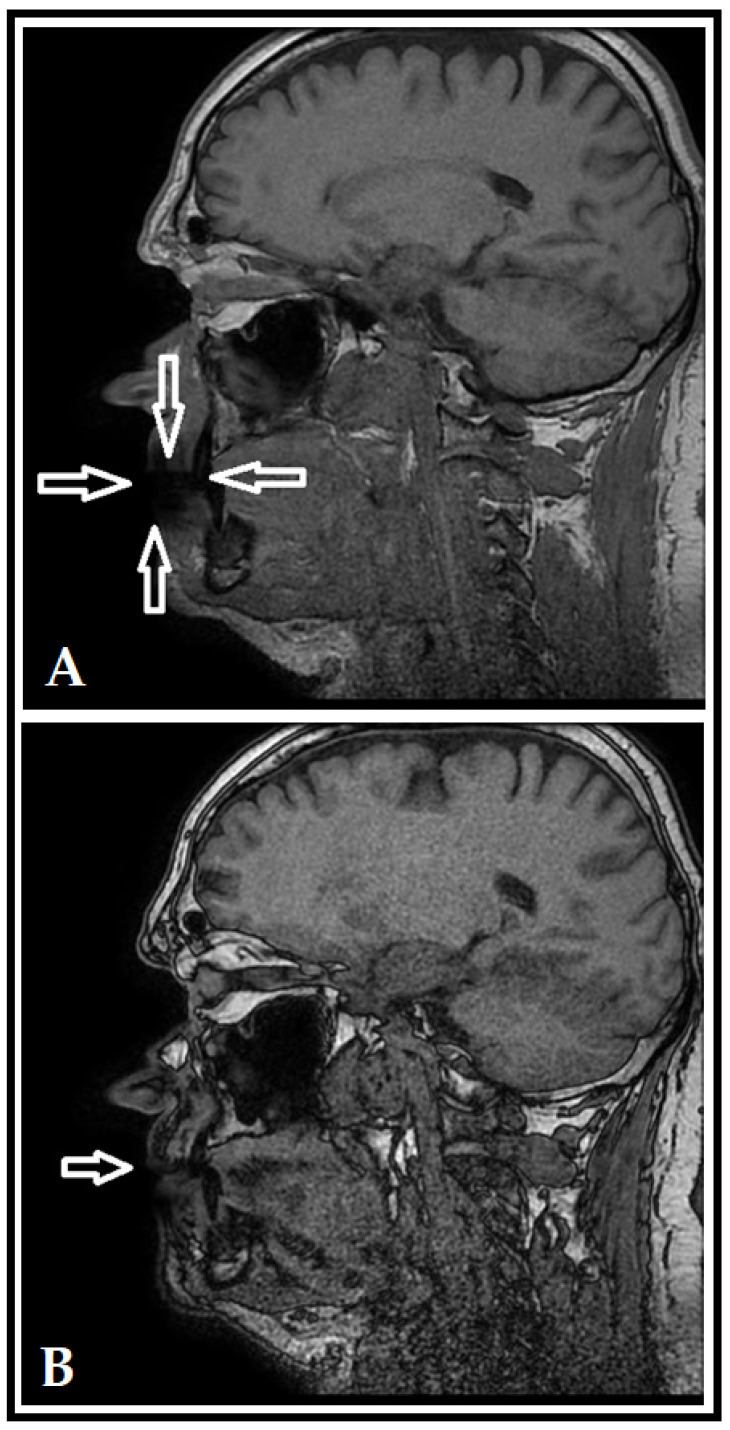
Unadjusted (A) and adjusted (B) MRI scans for Patient 2 (T1 sag). The shadow in the area of the lips (denoted by white arrows) indicates the imaging artefact caused by Cu accumulation in the lips, which is less pronounced after using adjusted directions and measurements.
